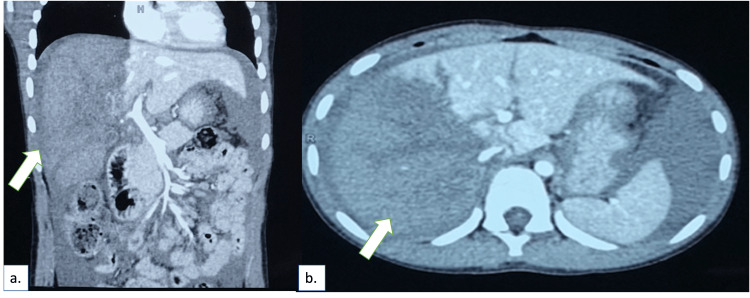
Contrast-enhanced computed tomography done at admission showing nonenhancing right lobe (arrow) with hemoperitoneum: (a) coronal section; (b) axial section.
